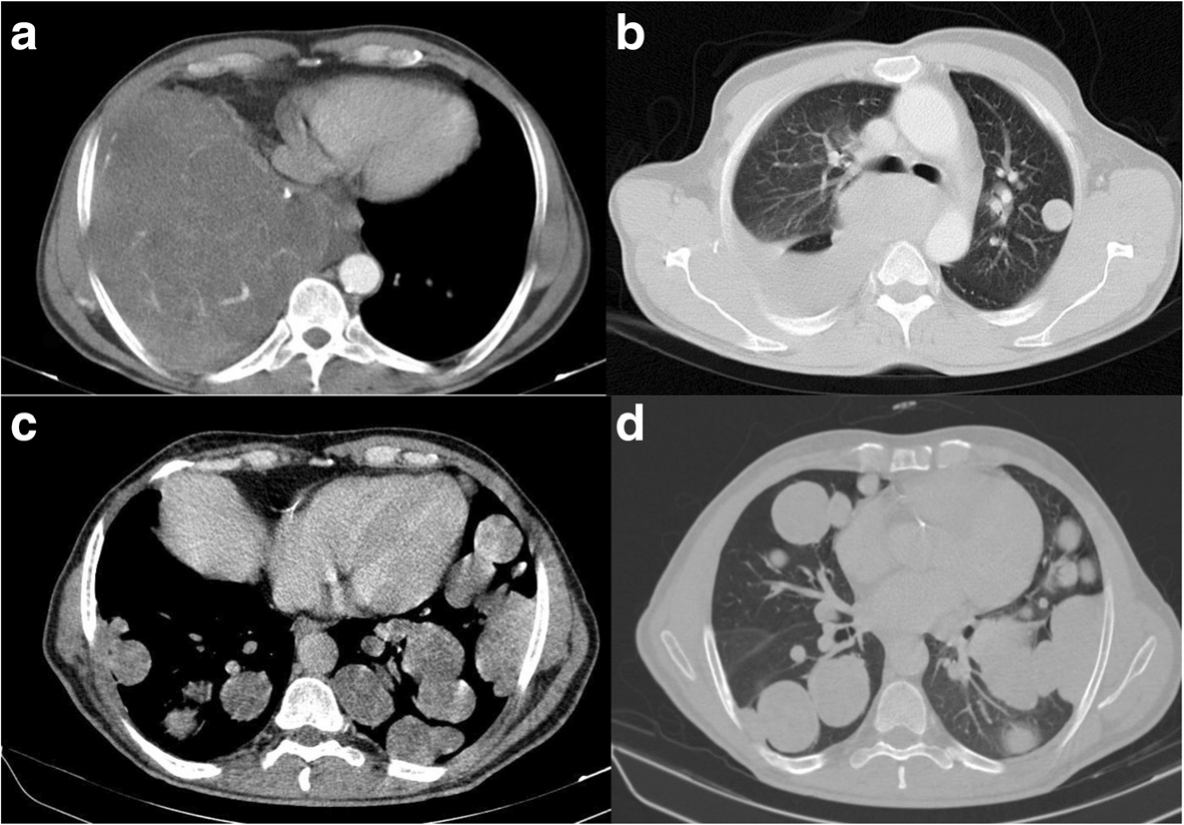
Contrast enhanced axial CT scan of the patient before a, b and after c, d surgery. There is a large solid mass in lower zone of right hemithorax with complete collapse of right lower lobe and cardiac displacement to left a. There is also an 18 × 15 mm parenchymal nodule in left upper lobe. Several other smaller nodules are found in this exam (not shown) in the rest of left lung. Two years after resection of the large mass in basal aspect of right hemithorax, there are numerous bilateral parenchymal and pleural-based nodules and masses with maximum size of 42 × 40 mm c, d
